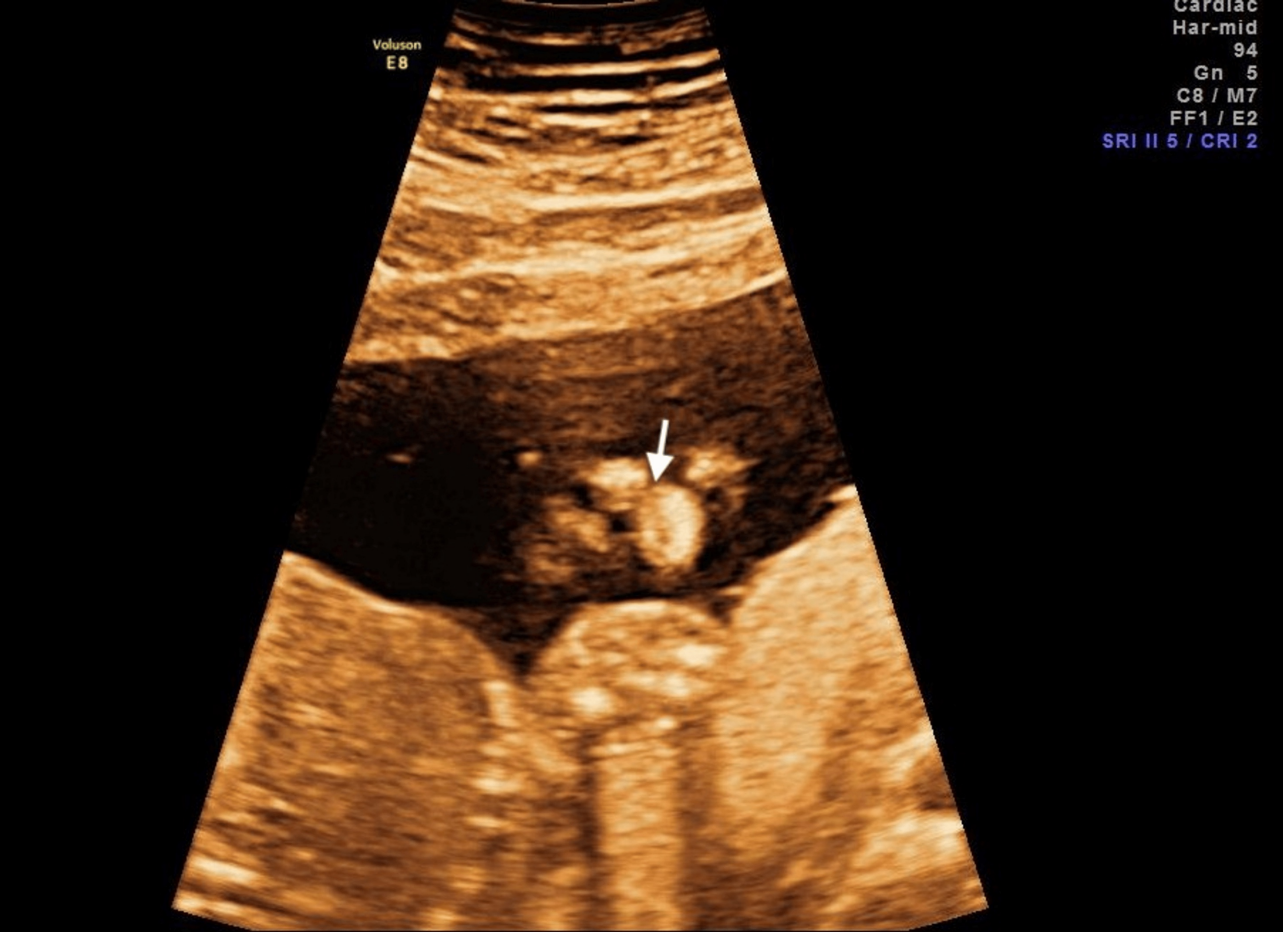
Unilateral pleural effusion at midtrimester ultrasound Right unilateral mild pleural effusion and cleft lip (white arrow) at midtrimester routine ultrasound (coronal view).
